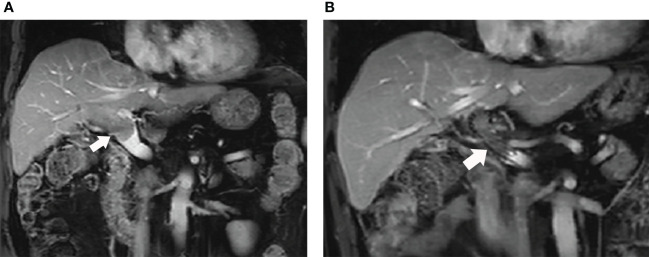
(A) The MRI images of this male patient shows the tumor thrombus had invaded into the MPV from right portal vein (white arrow); (B) The patient received ^125^I seed strands and PVS implantation plus TACE combined with lenvatinib and anti-PD-1 antibody therapy. The MRI images performed 11 months after the initial procedure shows the stent is still patent (white arrow).
